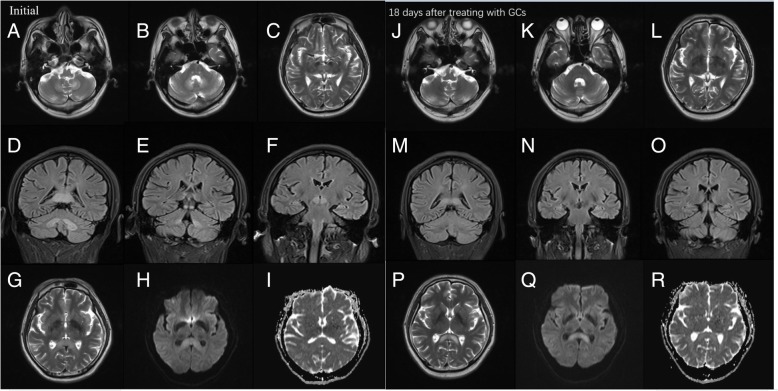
MRI findings. Scanning was performed by using 3.0 T MR imaging systems (Siemens). Initial MRI findings.T2W MRI images show hyperintensities in bilateral cerebellar dentate nuclei (a), medulla oblongata (a), midbrain (b) and red nuclei (c). Coronal FLAIR images show hyperintensity in bilateral dentate nuclei (d), splenium of the corpus callosum (d), gray matter near the midbrain ducts (e) and red nuclei (f). Moreover, T2W image shows slight hyperintensity in splenium of corpus callosum (g), but DWI and ADC images only show diffusion restriction in the corresponding areas of corpus callosum (h, i). Follow-up MRI imaging was conducted 18 days after treating with GCs (22 days after discontinuation of metronidazole). T2W images (j-l) and coronal FLAIR images (m-o) show complete resolution of the previously noted signal changes. However T2W image shows higher hyperintensity in splenium of corpus callosum in axial (p). DWI and ADC images show diffusion restriction and a high ADC respectively in the corresponding areas of corpus callosum (q, r)
